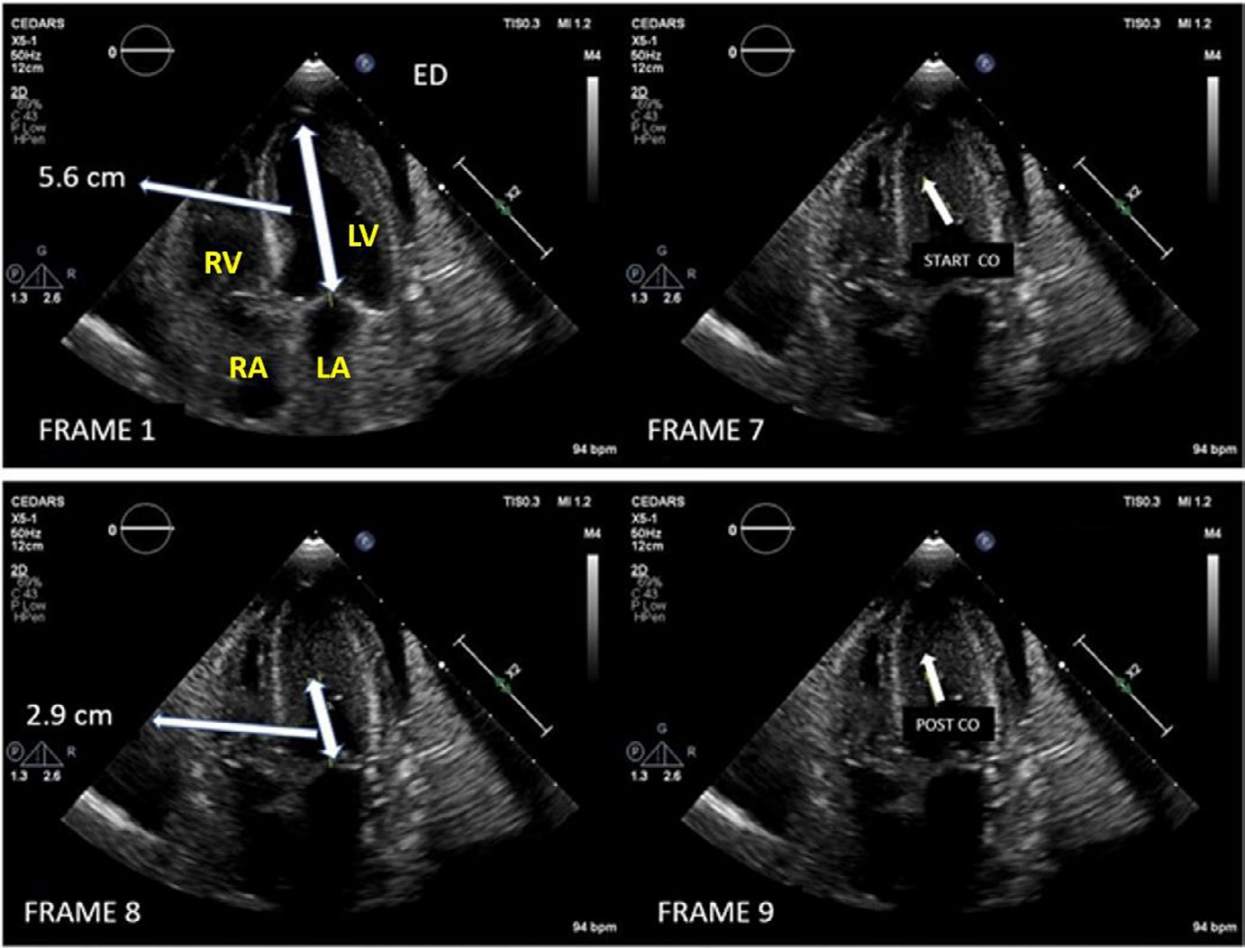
2D echo apical 4‐chamber frames in a LVCO patient with peak ICG of 2.1 mm Hg. End‐diastolic (ED) frame at top left. Apical length from apex to mitral annulus is 5.6 cm. Cavity obliteration first occurs in frame 7 and has already ended by frame 9 (post‐CO), which shows a tiny gap between the LV walls; therefore, only 2 frames; 7 and 8: are obliterated. At a frame rate of 50 Hz, this equals 20 ms per frame, and therefore, obliteration lasts 2 x 20 ms, or 40 ms. End obliteration apex to annulus length in frame 8 is 2.9 cm. Percent obliteration = 5.6‐2.9/2.9 = 48%
